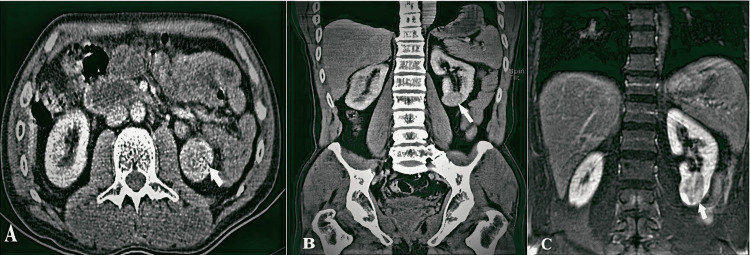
Eeosinophilic vacuolated tumor in a 47-year-old man A-B: Computed tomography transverse and coronal sections showing well-defined round heterogenous enhancement of the left lower pole renal mass (white arrows); C. Coronal contrast-enhanced T1-weighted image shows heterogeneous enhancement in the left lower pole renal mass (white arrow).
